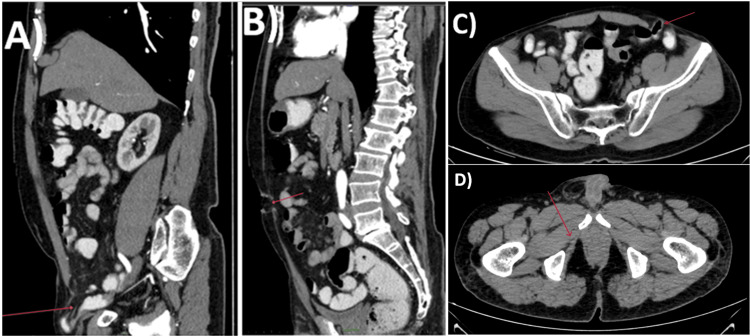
CT scan abdomen and pelvis. A) The sagittal section of the contrast-enhanced computed tomography (CECT) of the abdomen and pelvis shows a right inguinal hernia containing an ileum loop and omentum as its contents. B) Sagittal section of CECT of the abdomen and pelvis showing a defect measuring 12 x 10 mm (craniocaudal x transverse) in the anterior abdominal wall in the umbilical region within the linea alba, with herniating omentum as its content, suggestive of an umbilical hernia. C) Axial section of CECT of the abdomen and pelvis showing a defect measuring 52 x 35 mm (craniocaudal x transverse) in the anterior abdominal wall in the left iliac region at the lateral border of the rectus abdominis muscle, with herniating proximal sigmoid colon loop and mesentery as contents, suggestive of a left Spigelian hernia. D) Axial section of CECT of the abdomen and pelvis showing the right obturator foramen.
